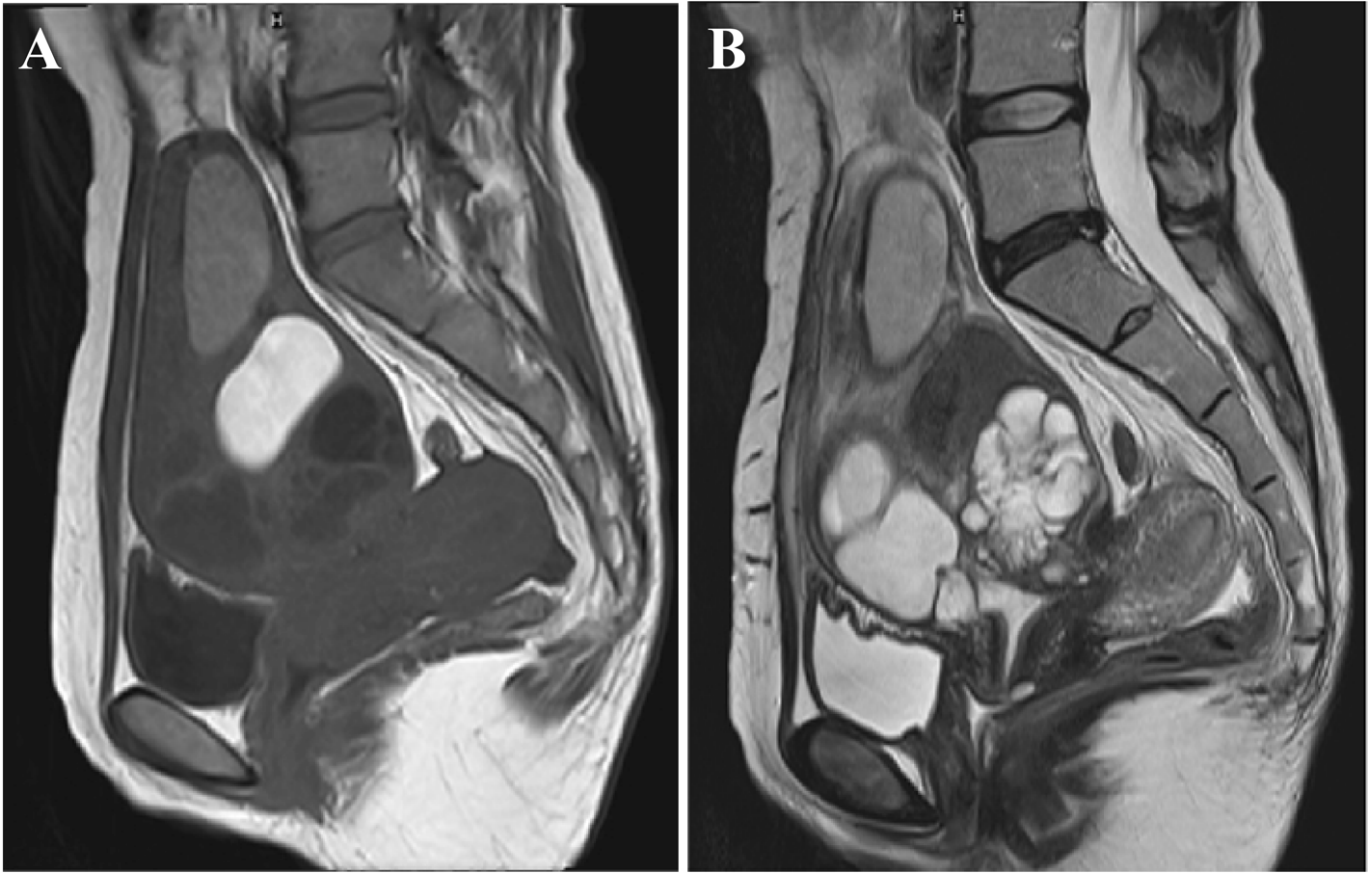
Magnetic resonance imaging (MRI) of the pyogenic cervical cyst. (A) a sagittal T1-weighted and (B) a sagittal T2-weighted imaging revealed a large cystic mass at the anterior cervix. The mass was a complex multilocular cyst, consisting of at least two components. The cranial part of the cyst contained two high-intensity cystic masses on the T1-weighted image, and the caudal part contained two multilocular masses that demonstrated low-intensity on the T1-weighted image and high-intensity on the T2-weighted image.
